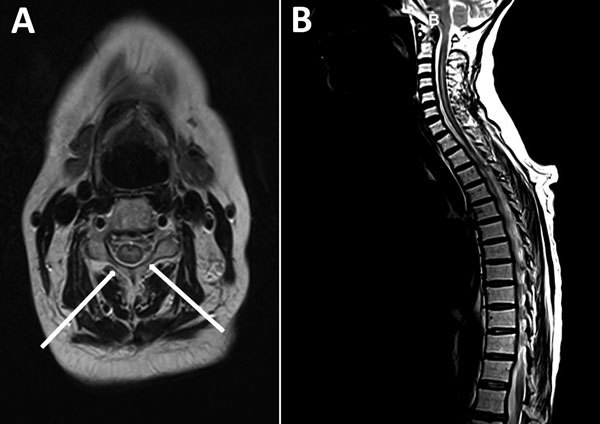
Magnetic resonance images of the brain and spinal cord of a woman who later died of fatal neurologic disease associated with enterovirus D68 infection. A) Sagittal and axial T2 image of the spinal cord showing cord swelling, particularly at the cervical level (arrows). B) Extensive hyperintensity in the central cord.
